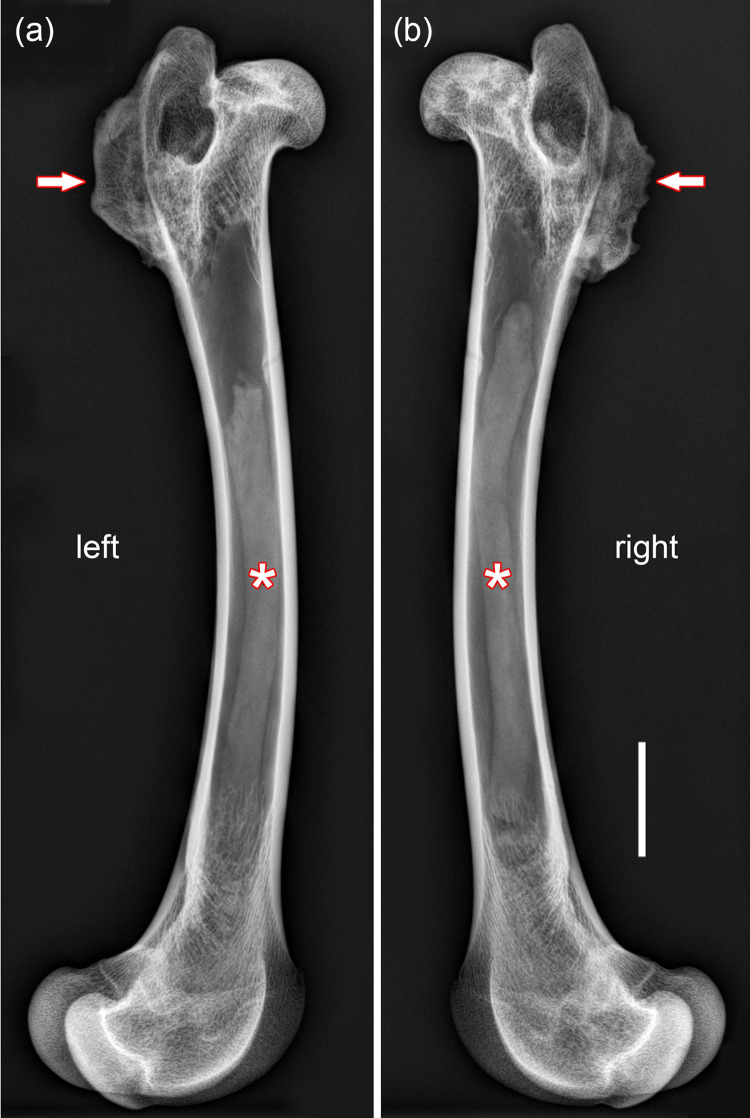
Radiographs (mediolateral projections) of the pathological femora of the roe buck; bar in (b) equals 20 mm and applies to both images. (a) Left femur. (b) Right femur. Arrows: Bony outgrowths in the regions of the intertrochanteric crest. Asterisks: Objects in marrow cavity.
